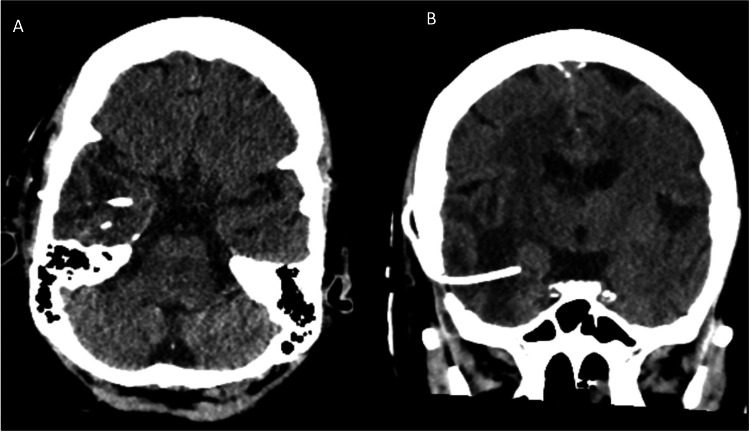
Post-operative imaging. Axial (A) and coronal (B) view of post-operative CT demonstrating placement of Ommaya reservoir.
